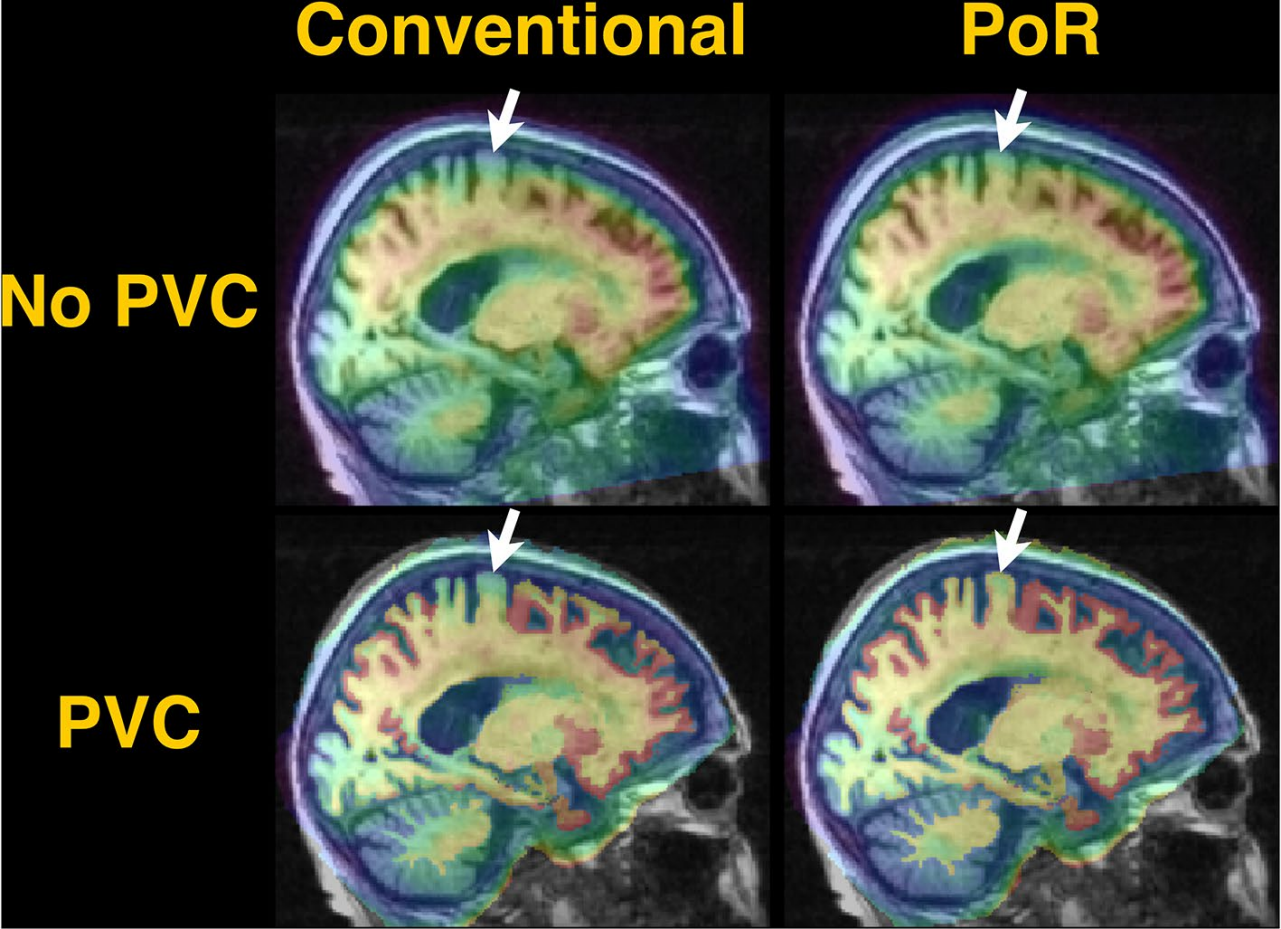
SUV images, fused with a MR T1-weighted image, without PVC (upper row) and with PVC (lower row) in a case with the largest translation on the *z*-axis (an 83-year-old female patient with MCI). Results are derived from using the conventional registration (left) or PoR (right). Thus, images on upper left, upper right, lower left and lower right indicate PV-uncorrected SUV maps registered with the conventional and PoR methods and PV-corrected SUV maps registered with the conventional and PoR methods, respectively. Differences between the two methods in this case were 0.32, 0.65, and 2.74 mm for translations in the *x-*, *y-*, and *z*-axes, respectively, and 3.02°, 0.34°, and 0.35° for rotations in the *x-*, *y-*, and *z*-axes, respectively. White arrows indicate parietal regions where remarkable differences in SUV between the conventional and PoR methods were observed
